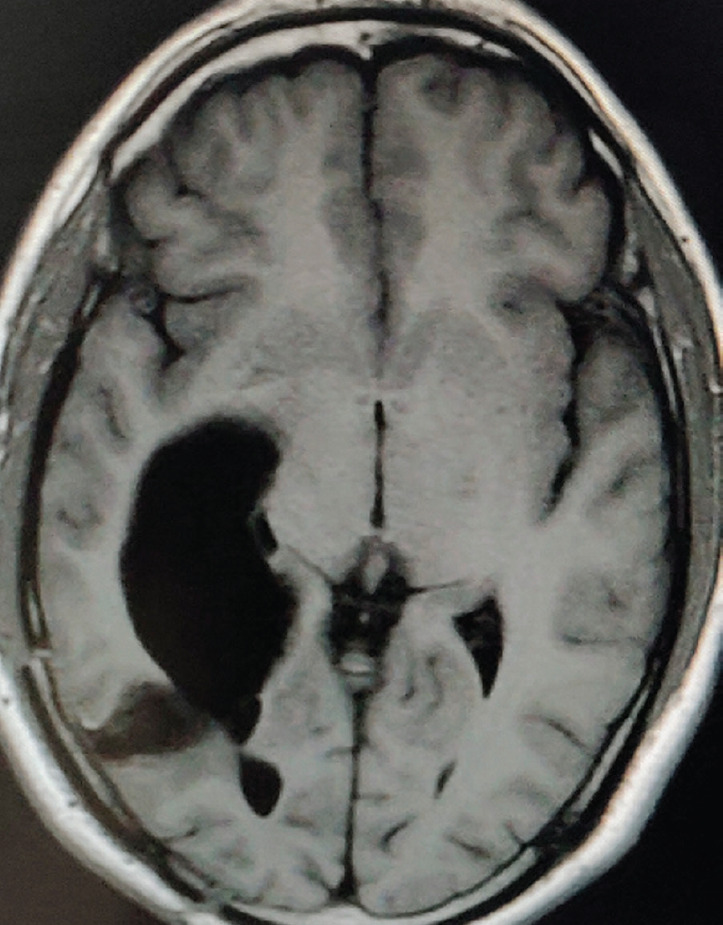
Post-op MRI Brain, T1W axial section showing a well-defined lobulated non-enhancing cystic lesion in right parietal lobe communicating with right lateral ventricle with dilated occipital horn.
